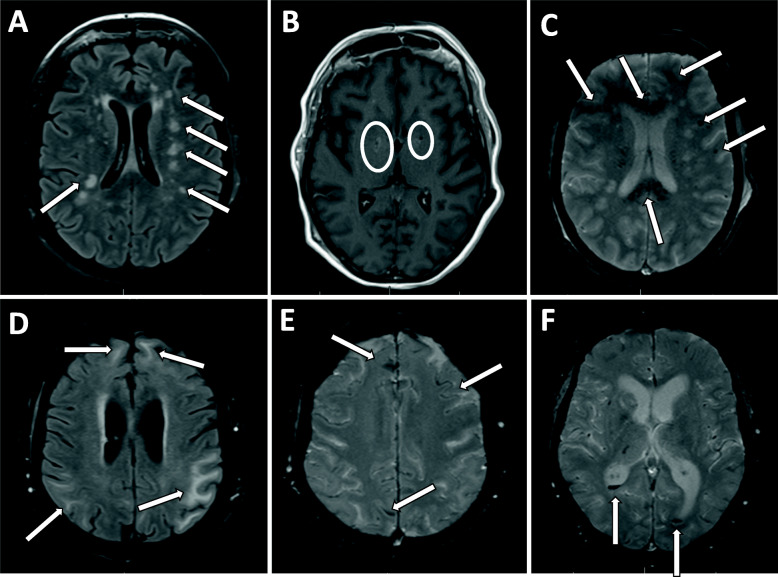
Exemplary MR imaging with multiple spotted (a) or confluent (b) hyperdense lesions (arrow) on axial fluid-attenuated inversion recovery (FLAIR) partly with contrast enhancement (circle) in the basal ganglia (b). Furthermore, evidence of multiple microbleeds in T2* sequences with emphasis on the corpus callosum (c) and the white matter as well as multiple cortical sulcal hemorrhages (e) and proof of ventricular hemorrhage (f). a-c 62 year-old female (patient #2) and d-f: 69 year-old male (patient #32), both with severe COVID-19 and acute respiratory distress syndrome
